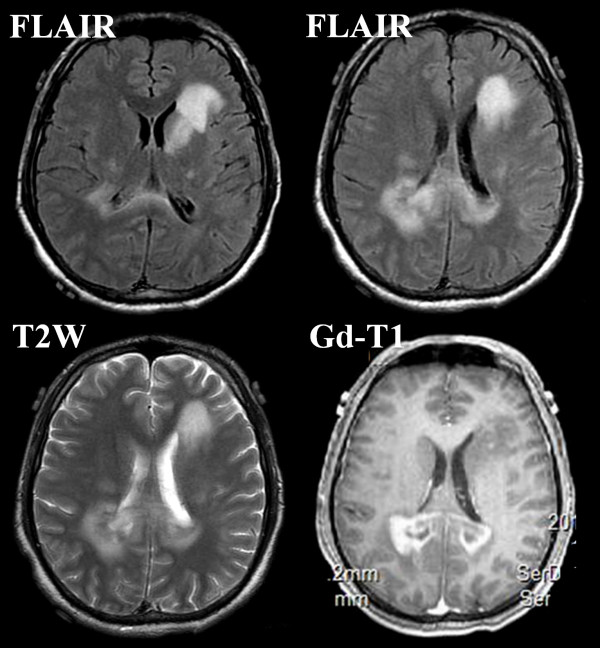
Magnetic resonance imaging after steroid pulse therapy. High-intensity areas in the left caudate head, bilateral semicentral ovales, and bilateral occipital forceps majors are clearly enlarged on FLAIR and T2W imaging. Gd-enhanced lesions in the occipital forceps majors are clearly expanded.
